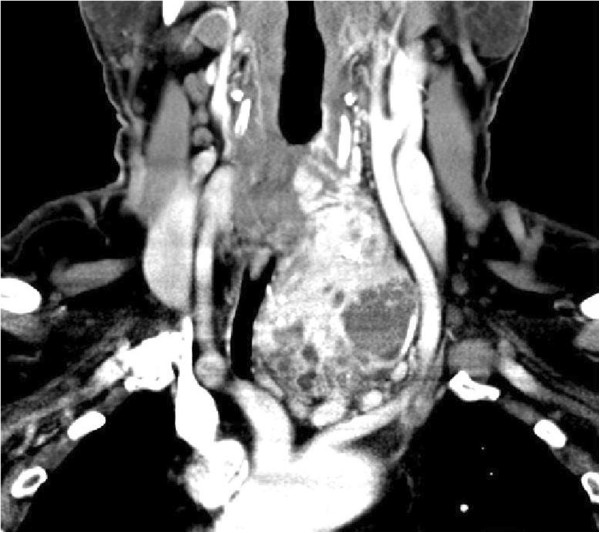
Computed tomography of the neck-coronal. Bulky left thyroid gland lobe displacing the branching arteries of the aortic arch and deviating the trachea to the right.
